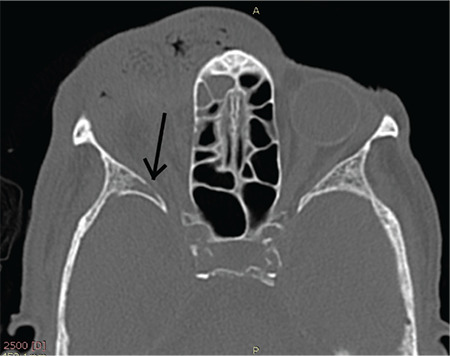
Computed tomography image showing bony erosion of the greater wing of sphenoid of the right orbit caused by *Chrysomya bezziana* larvae
